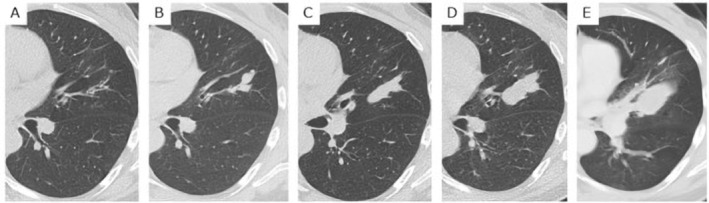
Chest computed tomography scan showed bronchiectasis in the lingual region of the left lung at the initial examination (A). One year later, a mucus plug appeared at the site of bronchiectasis (B). The mucus plug enlarged gradually at 3 years (C), 7 years (D), and 10 years (E) after the initial examination.
